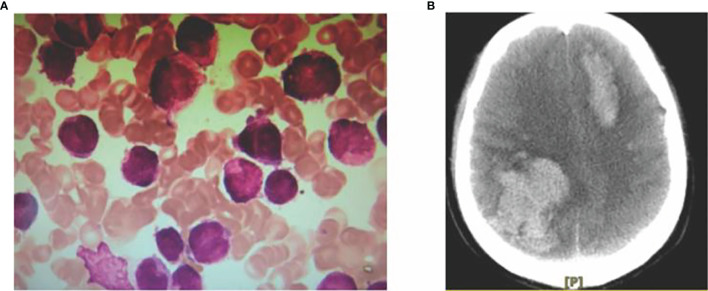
Myeloid morphology (A) and CT signs of intracranial hemorrhage in acute promyelocytic leukemia (B).
